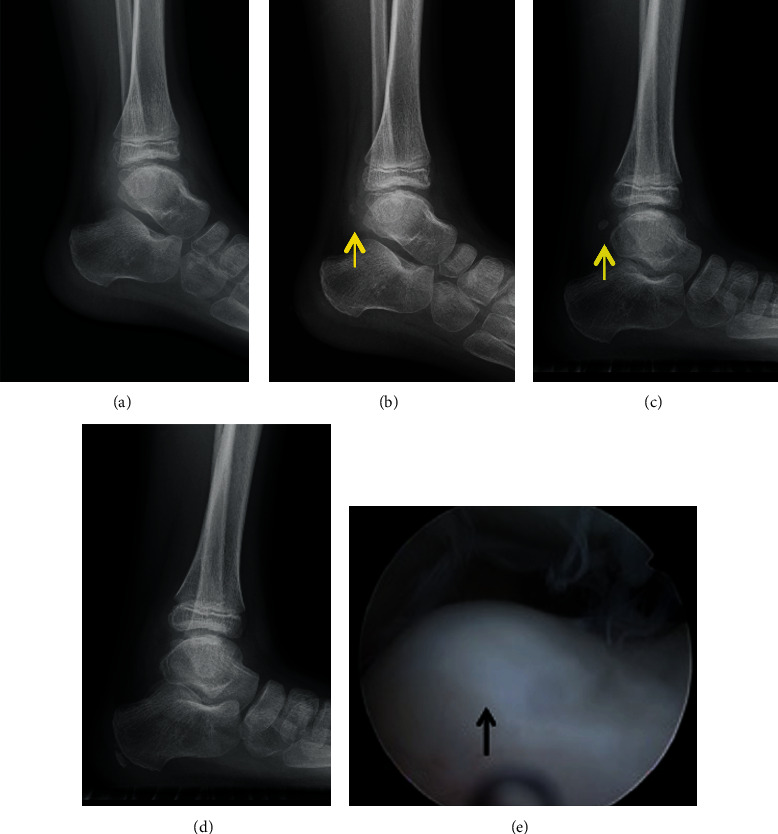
Chronological ankle lateral plain radiograph. (a) Postoperative three months after primary surgery, (b) postoperative five months after primary surgery, (c) postoperative one year after primary surgery, and (d) postoperative six months after secondary surgery. (e) Arthroscopic findings during secondary surgery. Gradual growth of a new oval ossified free body in the posterior joint space of his left ankle after primary surgery (yellow arrow) (a–c). No recurrence after secondary surgery after six months postoperatively (d). The cartilage defect due to resection of the protuberance from the talus, which was treated with microfracture technique during primary operation, was covered with healthy cartilage (black arrow).
